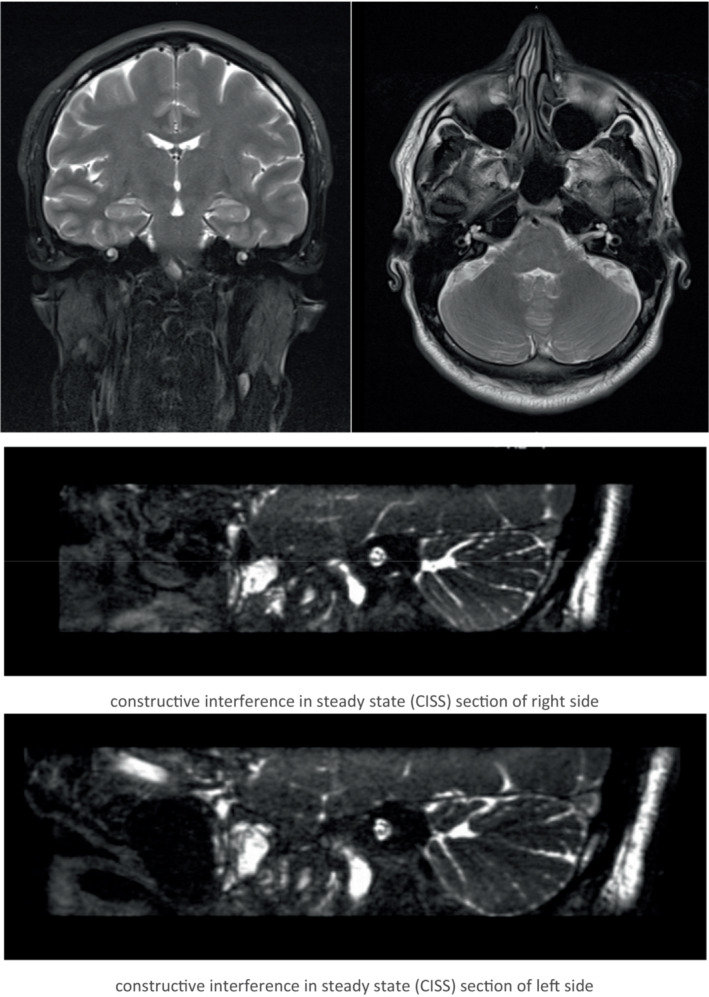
MRI sections of head and internal auditory meatus.
